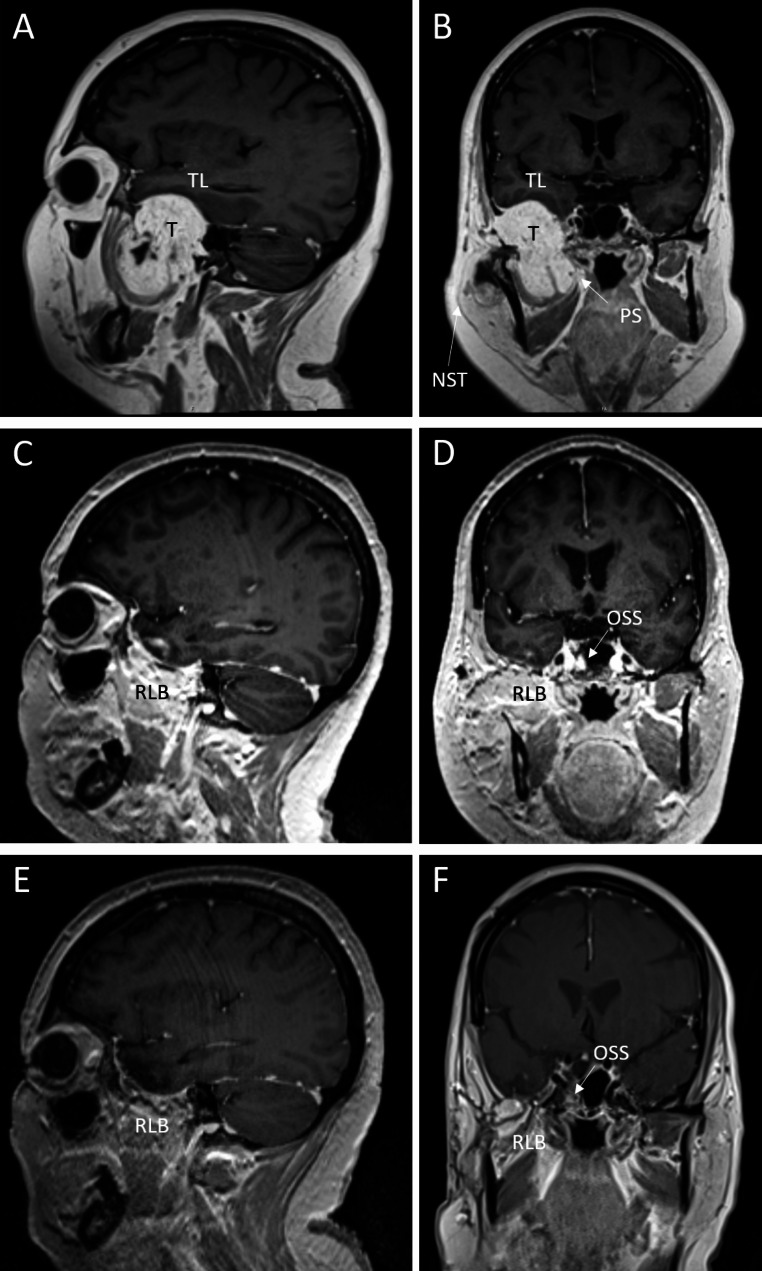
MRI scan. T1 with contrast, A and B: tumor extension preoperatively. Tumor (T) infiltrating the temporal and infratemporal fossa, parapharyngeal space (PS) and the middle fossa. Mild upwards compression of the right temporal lobe (TL). Asymmetry of the neck soft tissue (NST). C, D: 4 months postoperatively and E, F: 2 years and 4 months postoperatively no evidence of residual tumor and recurrence after reconstruction of the laterobase (RLB) and partial occlusion of the sphenoid sinus (OSS) with autologous fat. A, C, E: sagittal. B, D and F: coronar
